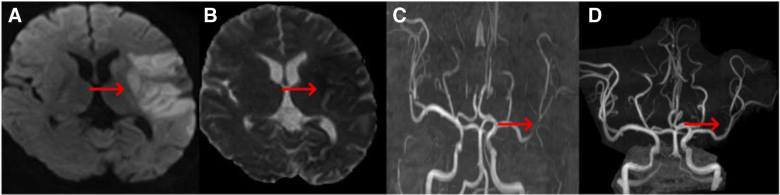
Admission and 12-month follow-up neuroimaging. (A) Axial DWI demonstrates hyperintense infarcts in the left temporal, basal ganglia, and parietal regions, with (B) corresponding hypointensity on the ADC map. (C) Baseline MRA reveals moderate-to-severe stenosis of the left MCA M2 segment with attenuation of distal branches. (D) Follow-up MRA at 12 months shows luminal recovery at the prior M2 stenotic segment. ADC = apparent diffusion coefficient, DWI = diffusion-weighted imaging, MCA = middle cerebral artery, MRA = magnetic resonance angiography.
